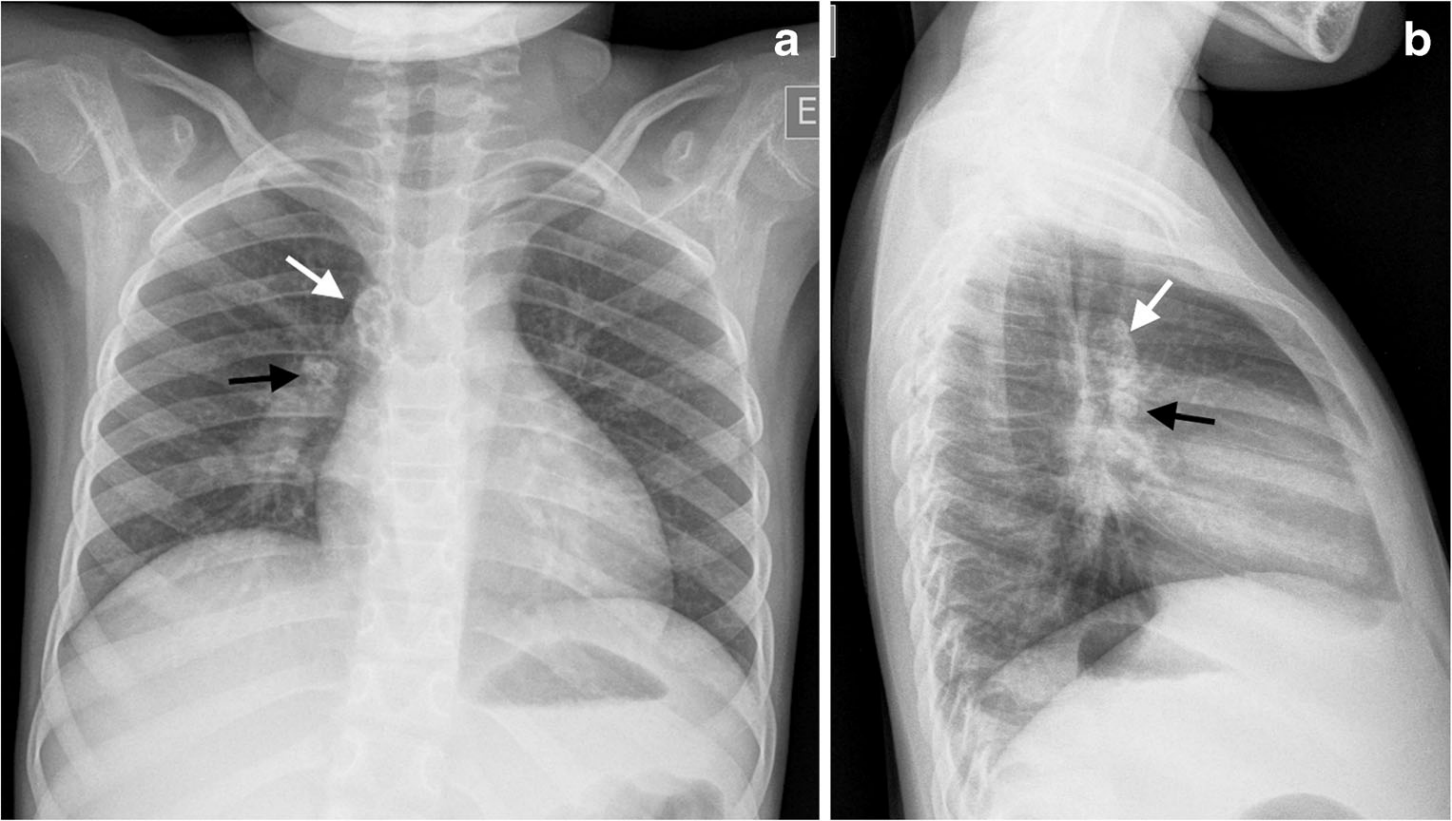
Imaging in an 8-year-old girl at a Médecins Sans Frontières site in the Democratic Republic of Congo referred for tele-reporting. Presenting symptoms were cough and fever, and the girl was not responsive to antibiotic treatment for suspected pneumonia. The request indicated that pneumonia and tuberculosis were being considered despite no known contact history. a Anteroposterior chest radiograph demonstrates calcified lymphadenopathy at the right paratracheal (*white arrow*) and right hilar regions (*black arrow*), consistent with primary pulmonary tuberculosis. b Lateral radiograph confirms the calcified paratracheal (*white arrow*) and hilar lymphadenopathy (*black arrow*)
